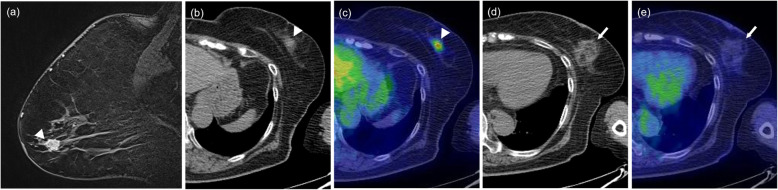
Fatty mass type. A 74-year-old woman with invasive ductal carcinoma. Breast MRI before cryoablation (a, arrowhead) showing a solid nodule of diameter 11 mm in the outer-lower quadrant of the left breast. The pre-cryoablation CT portion (b, arrowhead) and fusion image (c, arrowhead) of PET/CT show a solid nodule with abnormal FDG uptake (SUVmax, 3.53). On the CT portion (d, arrow) and (e, arrow) fusion image of PET/CT that was obtained 11 months after cryoablation, the treated area shows a focal fatty area and septa of soft tissue density with subtle FDG uptake (SUVmax, 1.34)
